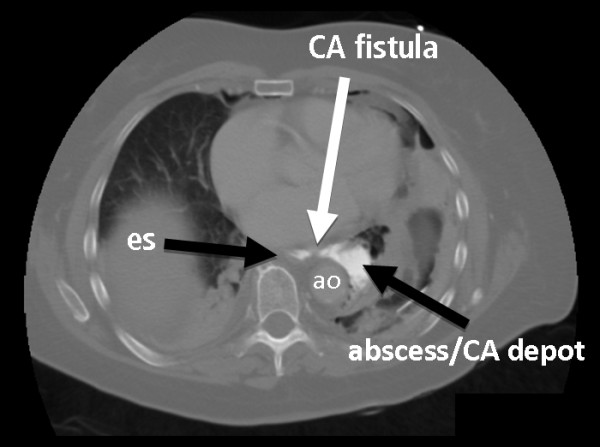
A computed tomography (CT) scan with oral contrast agent (CA) revealed only a thin CA line between the lumen of the esophagus and the mediastinal paraesophageal abscess/CA depot (es, esophagus; ao, aorta).
